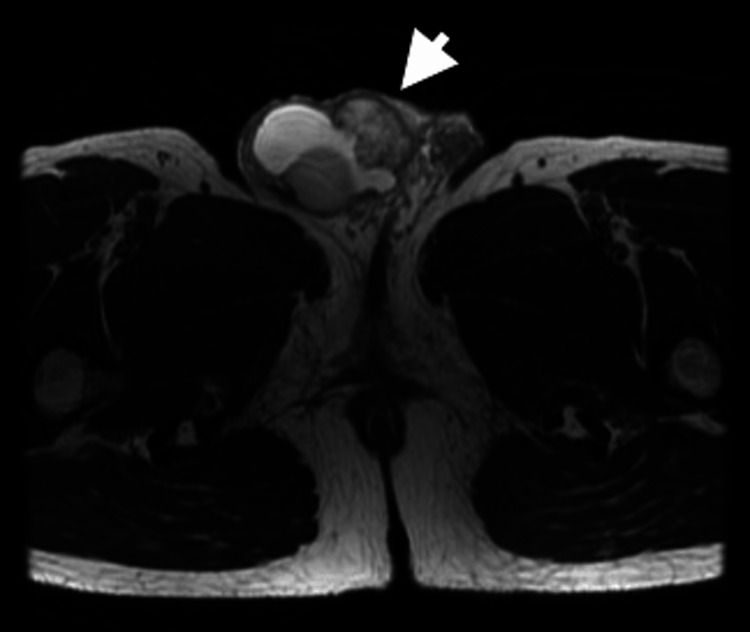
Magnetic resonance imaging (T2-weighted images) of the abdomen. Magnetic resonance imaging shows a tumor (arrow) with a low-intensity signal in the right spermatic cord.
